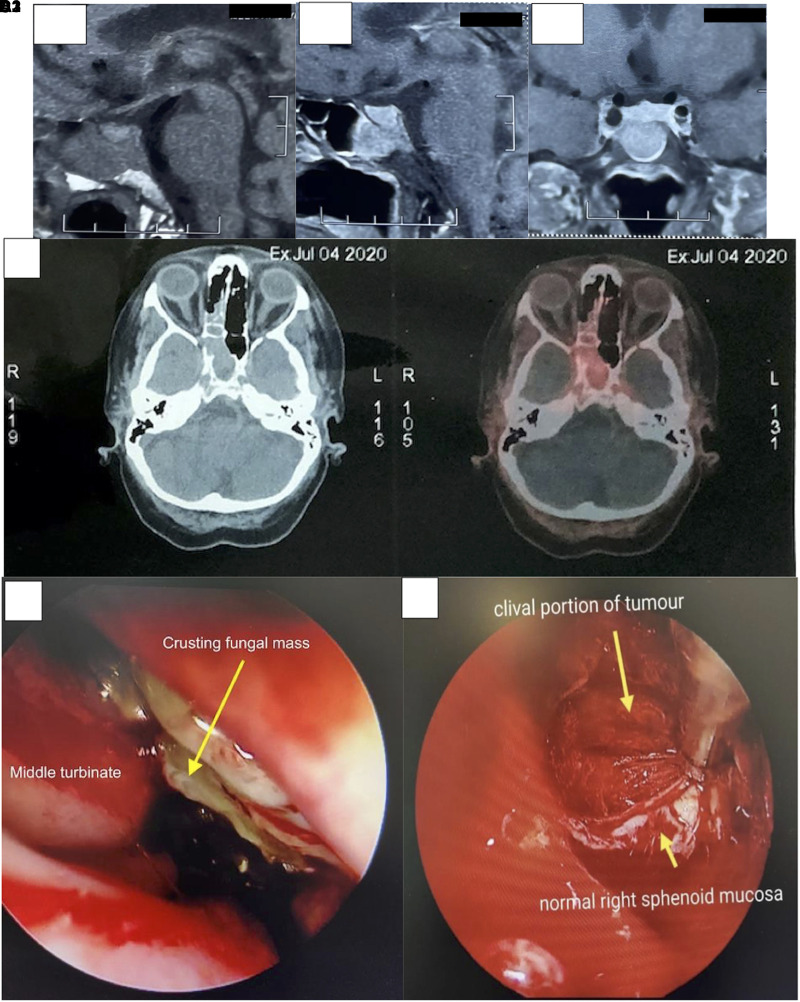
(A1) T1W MR of sella showing a normal sellar pituitary and heterogenous clival and right sphenoidal mass measuring 3 × 1.6 × 2 cm eroding the floor of sella. (A2, A3) Postcontrast sagittal and coronal cuts, respectively. (B) ^68^Ga DOTANOC PET-CT showing tracer-avid lesion in the right sphenoid sinus. Standardized uptake value maximum of the lesion was 4.7. (C) Incidentally detected crusting and eroding mass in the right nose, which was debrided and later turned out to be *Rhizomucor* spp. (D) Sphenoid sinus after debulking of the mass showing vascular clival tumor. Left sinus mucosa was seen as healthy tissue.
